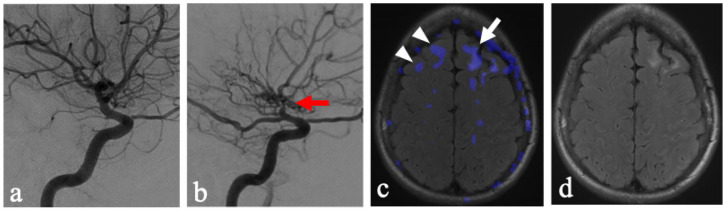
Adult patients with unilateral vaso-occlusive disease of the left anterior circulation. (a) A right-sided internal carotid artery DSA corresponding to the NFLH. (b) A left-sided internal carotid artery DSA corresponding to the FLH as evidenced by severe supraclinoid internal carotid artery stenosis (red arrow). (c) Axial T2/FLAIR MRI at the level of the centrum semiovale with areas of steal phenomenon overlaid in blue, demonstrating the occurrence of steal within the FLH (white arrow) greater than NFLH (white arrowheads). The steal grades for the right and left hemisphere were 1 and 3, respectively. (d) Axial T2/FLAIR MRI at the level of the centrum semiovale with T2/FLAIR hyperintensities in the left frontal lobe subcortical white matter. The Fazekas for the right and left hemisphere were graded independently as 0 and 2.
